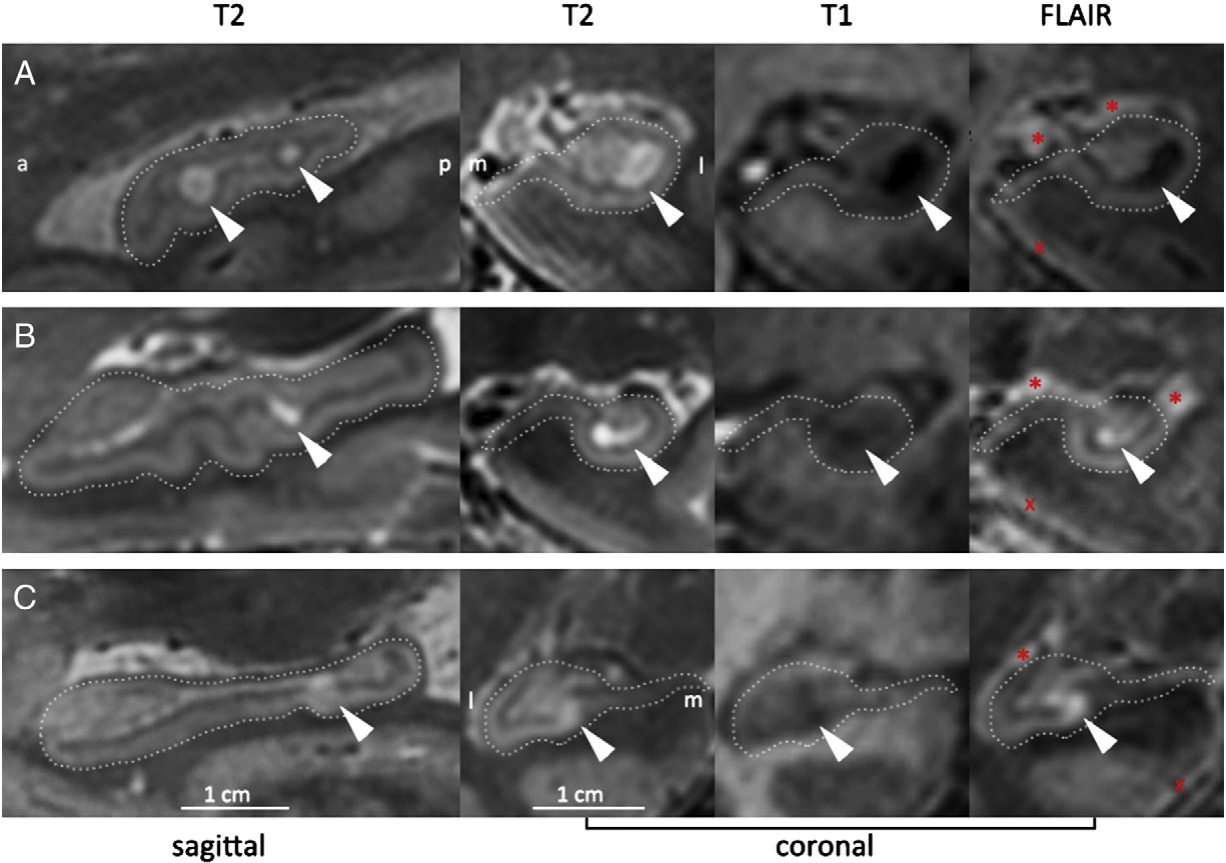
Hippocampal T2 hyperintensities on 7 Tesla MRI. Examples of hippocampal T2 hyperintensities that were hypointense (A) or hyperintense (BC) on 7 Tesla FLAIR. The left figure on each row is sagittal, the other three are coronal. For clarity, the hippocampus is outlined. Most HT2Hs appeared round or ovoid, but the ones that were hyperintense on FLAIR sometimes had a different shape. * indicates the choroid plexus, which is also hyperintense on FLAIR, but situated outside the hippocampus. Therefore it does not interfere with a reliable rating of focal HT2Hs within the hippocampus. X indicates the fluid — parenchyma border, which is also hyperintense on FLAIR. This hyperintense rim, characteristic for 7 Tesla FLAIR, is located along the outer surface of the brain and therefore does not interfere with a reliable rating of focal HT2Hs within the hippocampus. a: anterior; p: posterior; m: medial; l: lateral. The whole-brain 7 Tesla FLAIR scan of the participant whose hippocampus is depicted in panel C is available as a movie in the online supplementary material. Supplementary material Whole-brain FLAIR of a healthy 54-year old individual. This particular individual has a focal hippocampal FLAIR hyperintensity, possibly due to ischemia (best visible on sagittal view at 0:29min, and on coronal view at 0:34min). More details on 7T FLAIR contrast provided at [14]. This movie can be found at: http://www.youtube.com/watch?v=EaF_wbYlYc0.
